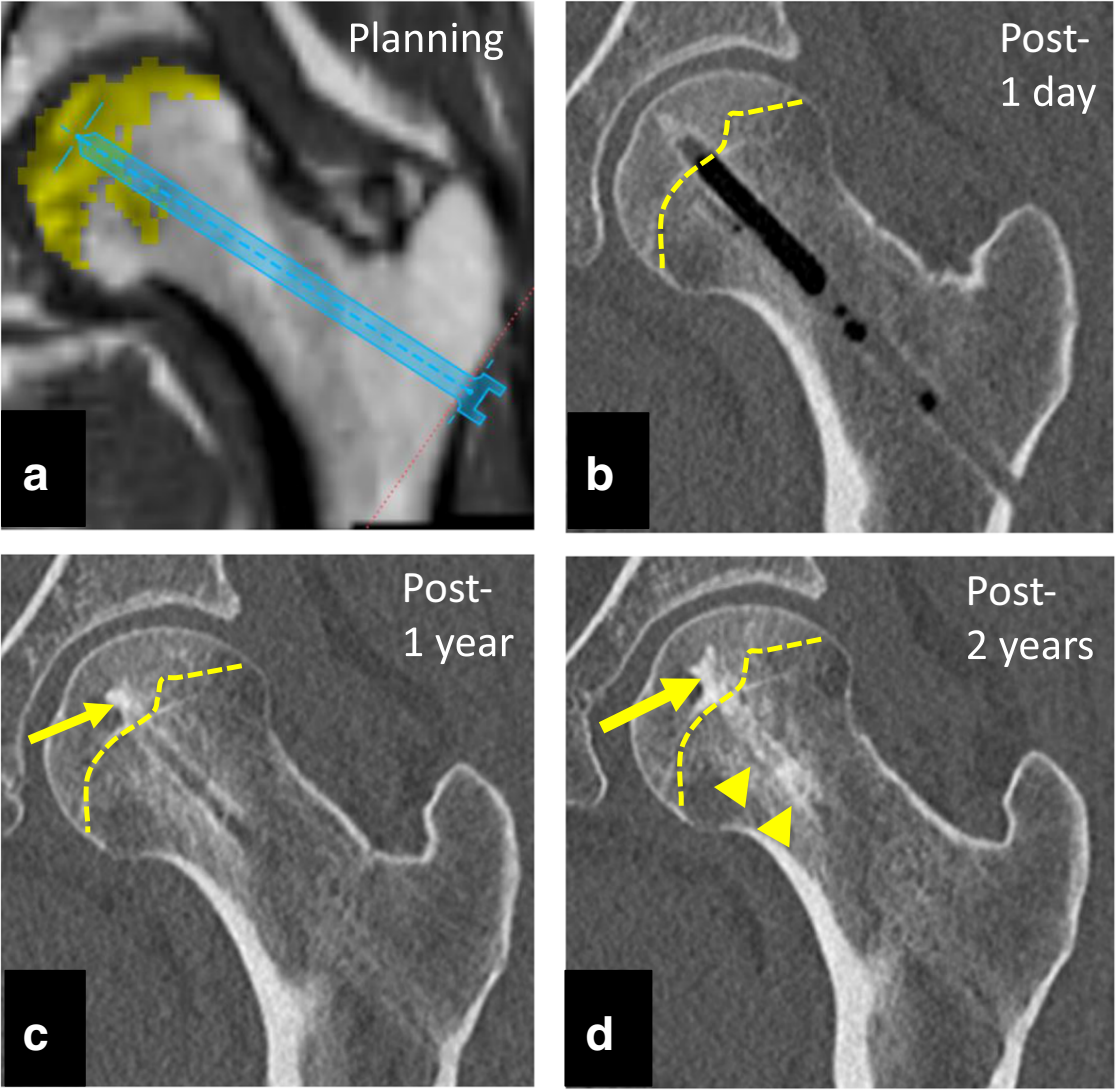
Planning and representative computed tomography images. a A screenshot of the preoperative planning. b Coronal computed tomography image shows a bone defect at the drilling route and implanted region 1 day postoperatively. The *yellow dashed line* shows the border of the osteonecrotic area of the femoral head. c In contrast, apparent bone regeneration of the osteonecrotic area is observed at 1 year postoperatively (*yellow arrow*). The normal contour of the femoral head is maintained. d Apparent bone regeneration of the osteonecrotic area is observed in the implanted region (*yellow arrow*) and drilling route (*yellow arrowheads*) at 2 years postoperatively. Normal contour, thick trabecular bone, and bone regeneration of the drilling route can be observed
